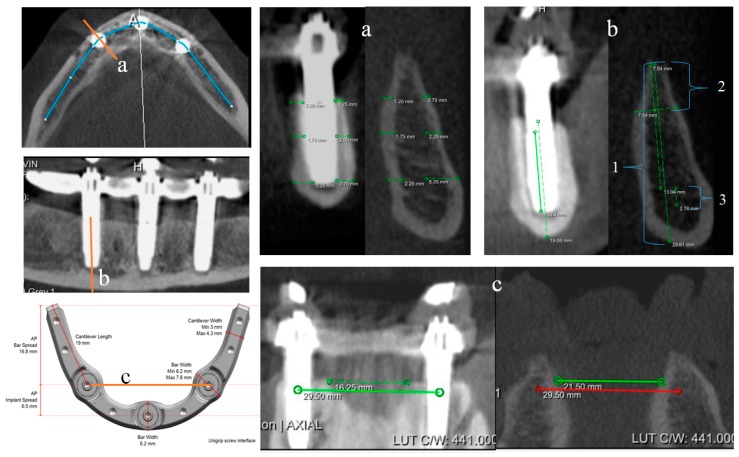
Measurements taken from CBCT images, on the reference model (left) and then transposed and adjusted on the test sample (right) (a) Buccal-lingual bone width at apex, middle and platform levels of each implant, (b) bone height measurements (1 = total bone height, 2 = bone resection height, 3 = distance from implant apex to incisive canal base), and (c) jawbone width measurements.
